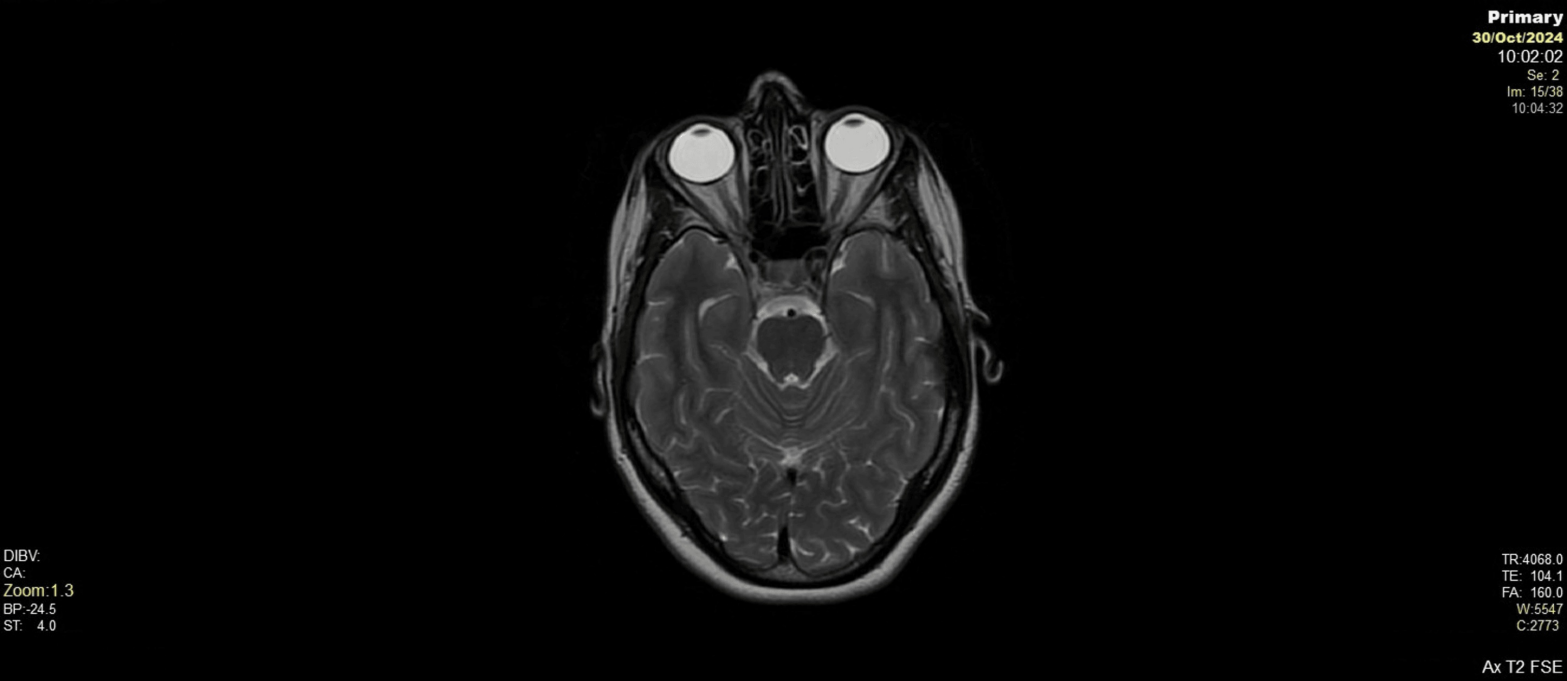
Brain axial T2-weighted MRI Axial T2-weighted MRI showing no central pontine demyelination, normal brainstem, cerebellum, temporal lobes, and basal cistern anatomy. No lesions, oedema, or structural abnormalities noted.
